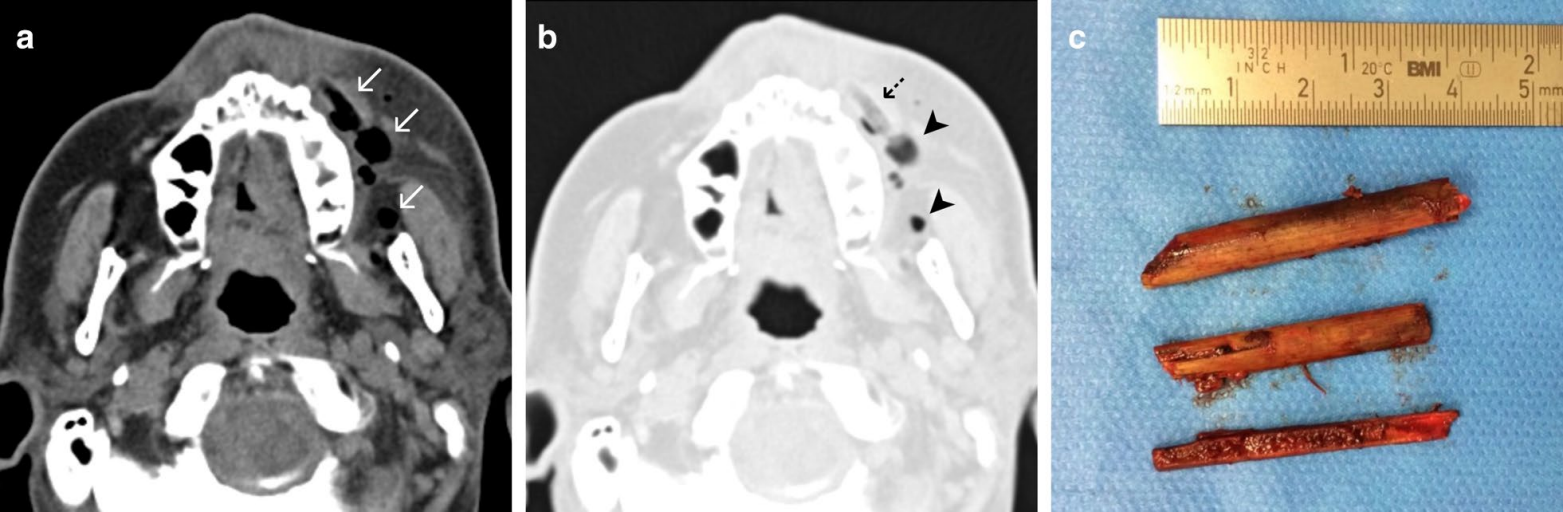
Wooden stick. Axial CT image in soft tissue window (a) shows phlegmonous fat stranding in the left buccal region, surrounding multiple hypodense features (arrows), giving the impression of emphysema. Closer inspection of the lung windows (b) reveals that one of the hypodense structures has a discernible internal structure (dashed arrow), distinguishing it from the homogenously hypodense gas locules (arrowheads). Three pieces of a wooden stick were surgically removed (c)
